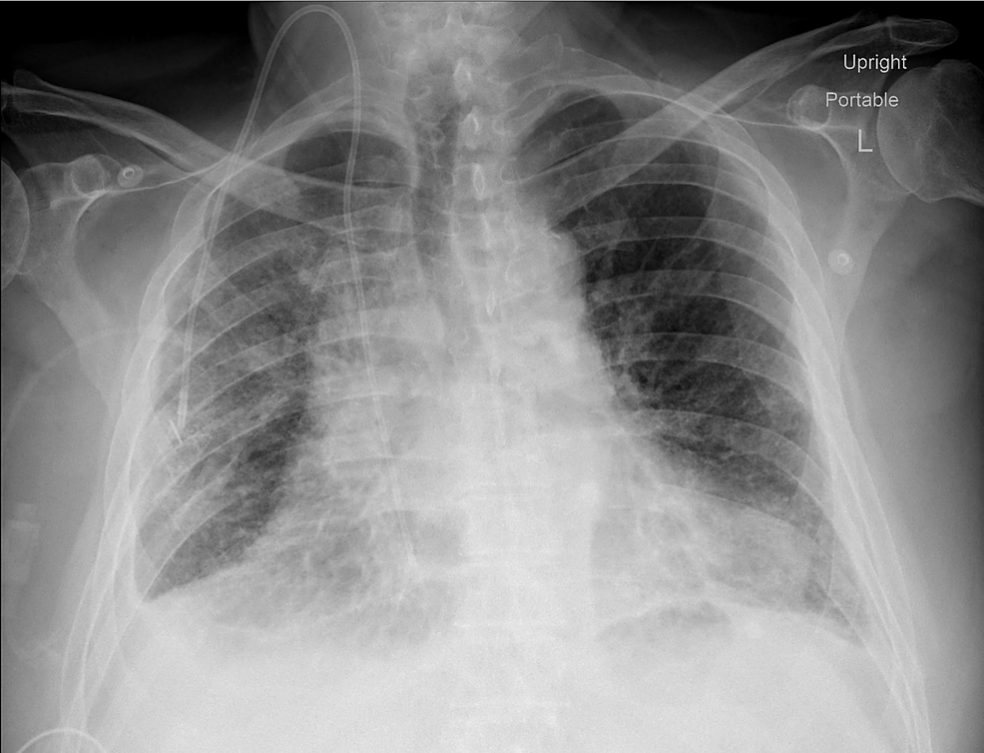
A chest radiograph antero-posterior view - poorly inflated lungs with bibasilar fibro-atelectatic changes and bilateral pleural thickening.
